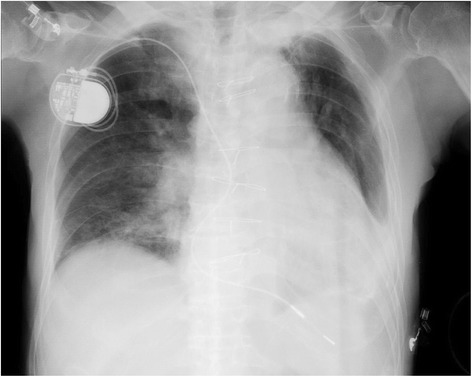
Portable supine chest radiograph in the operating room. It shows diffuse hilar haziness with increased density at the base of the right and left lungs. There were no signs for pneumothorax and a slight elevation of the right hemidiaphragm. Although the right hemidiaphragm was located at the fourth intercostal space, the right hemidiaphragmatic paralysis could not be diagnosed on this chest radiograph
